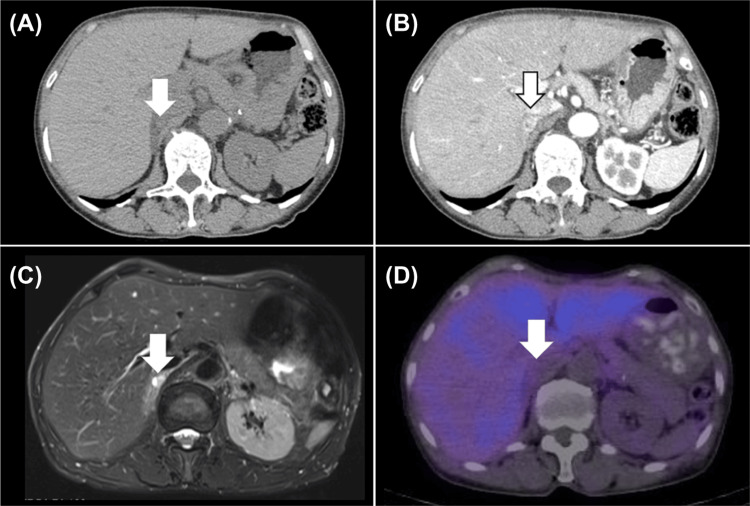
Imaging examination of the right adrenal tumor in case 31. (A) Plain CT. (B) Contrast-enhanced CT. (C) T2-weighted MRI. (D) 123I-MIBG scintigraphy. (A) Unenhanced CT image showing a small right adrenal mass. (B) Contrast-enhanced CT demonstrating mild enhancement of the lesion. (C) T2-weighted MRI revealing high signal intensity within the tumor. (D) ¹²³I-MIBG scintigraphy showing negative uptake in the right adrenal gland. ^123^I-MIBG: ^123^I-metaiodobenzylguanidine.
